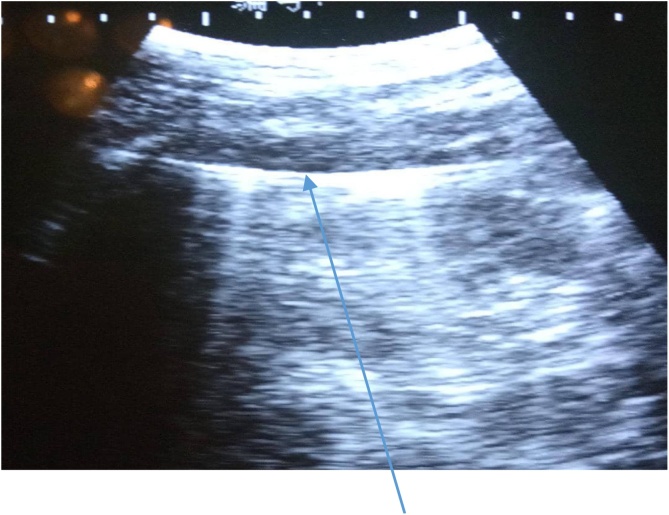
Two dimensional abdominal ultrasound scan image showing a curvilinear echogenic structure (Steinmann pin) with some posterior acoustic shadowing in the left lumbar region.
